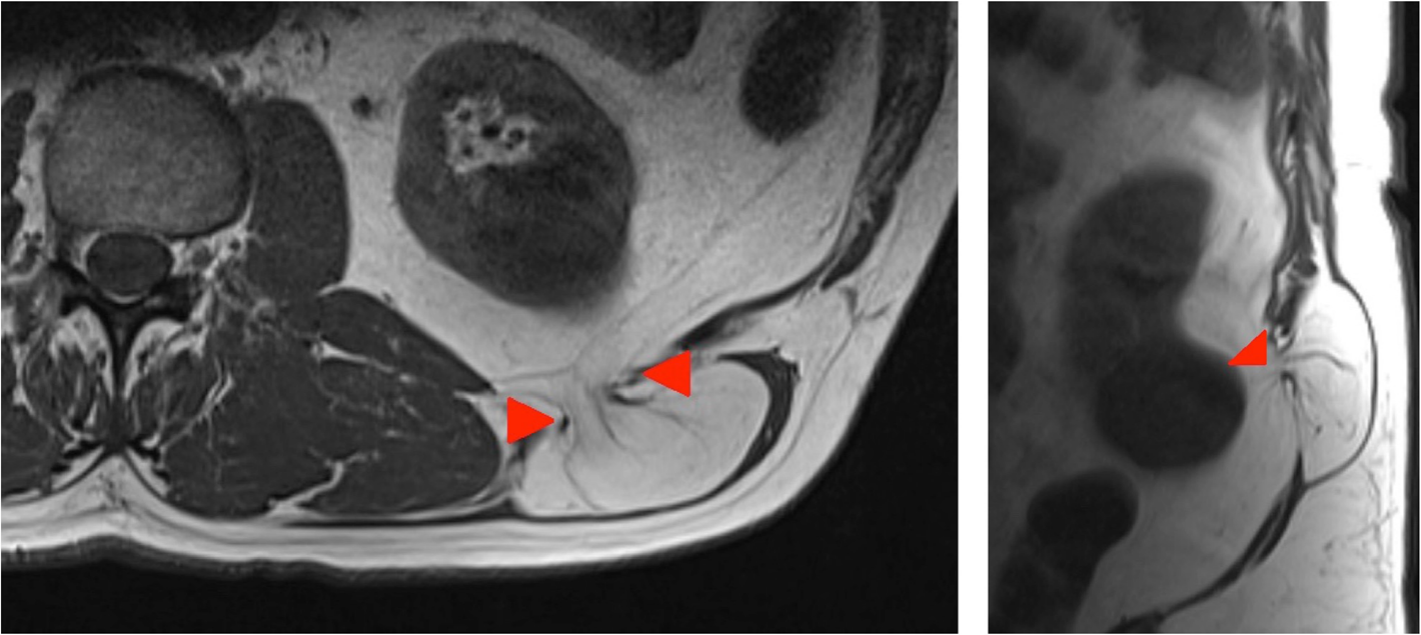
Left panel: Axial T1-weighted MR image demonstrates a defect in the left abdominal wall at the level of the inferior lumbar triangle, with protrusion of a retroperitoneal tumour with predominant fat signal intensity into the subcutaneous fat (red arrows). Right panel: Sagittal T1-weighted image: red arrowhead points out the hernial defect in the inferior lumbar triangle
